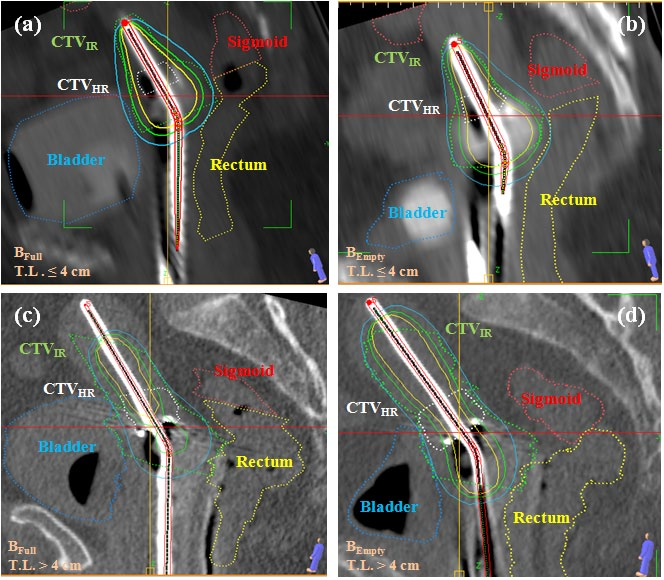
Contoured CT scan examples of two cervical cancer patients with inserted applicators: (a) and (b) tandem length<4 cm, and (c) and (d) tandem length<4 cm. Note: full bladder (a) and (c); empty bladder (b) and (d). Tandem length≠activation length in (c) and (d). (T.L.=tandem length, $B_{\text{Empty}}=\text{empty bladder}$, and $B_{\text{Full}}=\text{full bladder}$).
